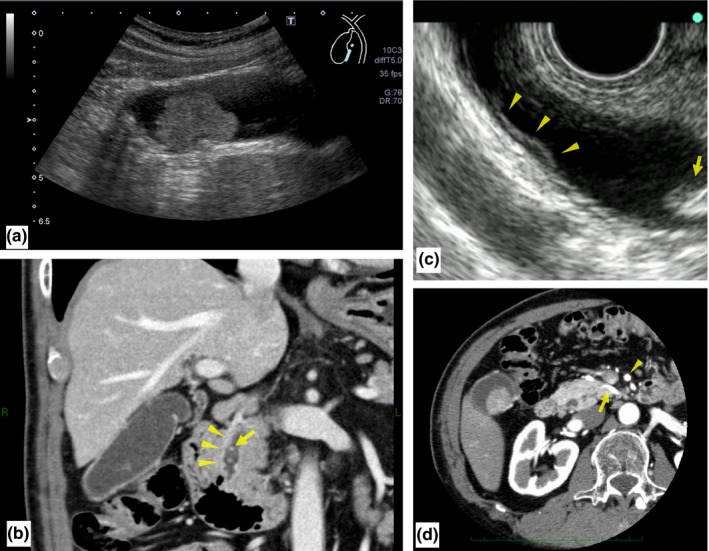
Preoperative images of the present patient. (A) Abdominal ultrasonography shows a papillary tumor in the gallbladder. (B) Abdominal computed tomography (CT) shows small stones (arrow) and the wall thickness of the intrapancreatic bile duct (arrowhead). (C) Endoscopic ultrasonography shows small stones (arrow) and mild irregularity of the mucosa in the lower bile duct (arrowheads). Combined with detection of adenocarcinoma by biopsy, this lesion was diagnosed as a T1 distal cholangiocarcinoma. (D) Abdominal CT shows the replaced common hepatic artery (arrow) originating from the superior mesenteric artery (arrowhead) and coursing along the ventral side of the pancreatic head parenchyma.
